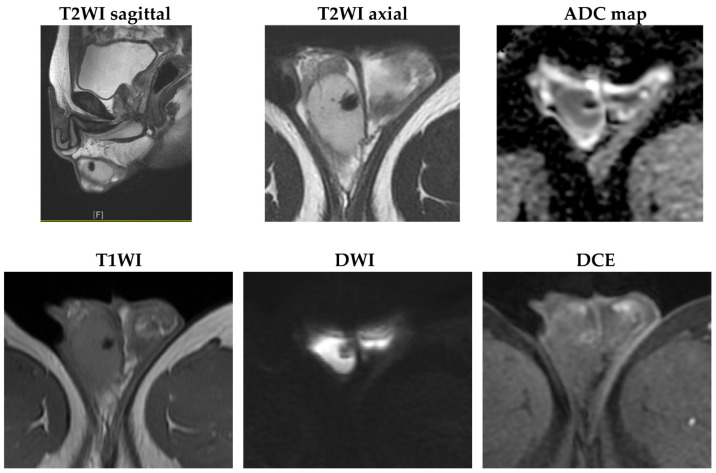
MRI images taken three months after the first attack revealed that the mass size had decreased to 7 mm. Hemosiderin deposits showed low signal intensity on all sequence images, with a rim-like reactive enhancement and reactive hydrocele (T2WI: T2-weighted image; ADC: apparent diffusion coefficient; T1WI: T1-weighted image; DWI: diffusion-weighted imaging; DCE: dynamic contrast-enhanced).
